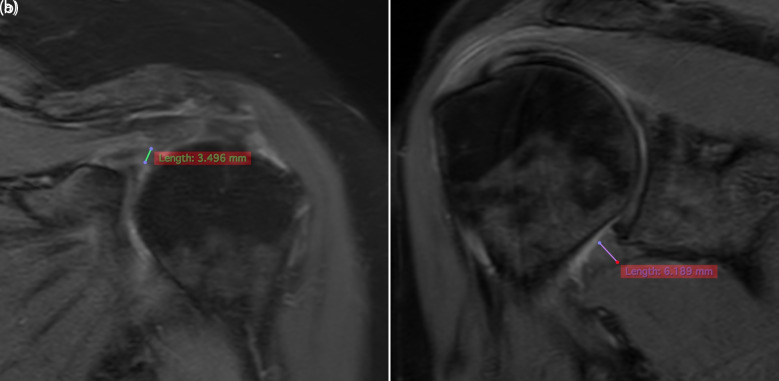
a. Measurement of the thickest slice of the coracohumeral ligament (CHL) in the coronal T2-weighted fat-suppressed MR image at the rotator interval level (3.49 mm). b. Measurement of the inferior glenohumeral capsule (IGHC) thickness at the axillary recess level in the coronal T2-weighted fat-suppressed MR image from the thickest edematous slice (6.1 mm).
